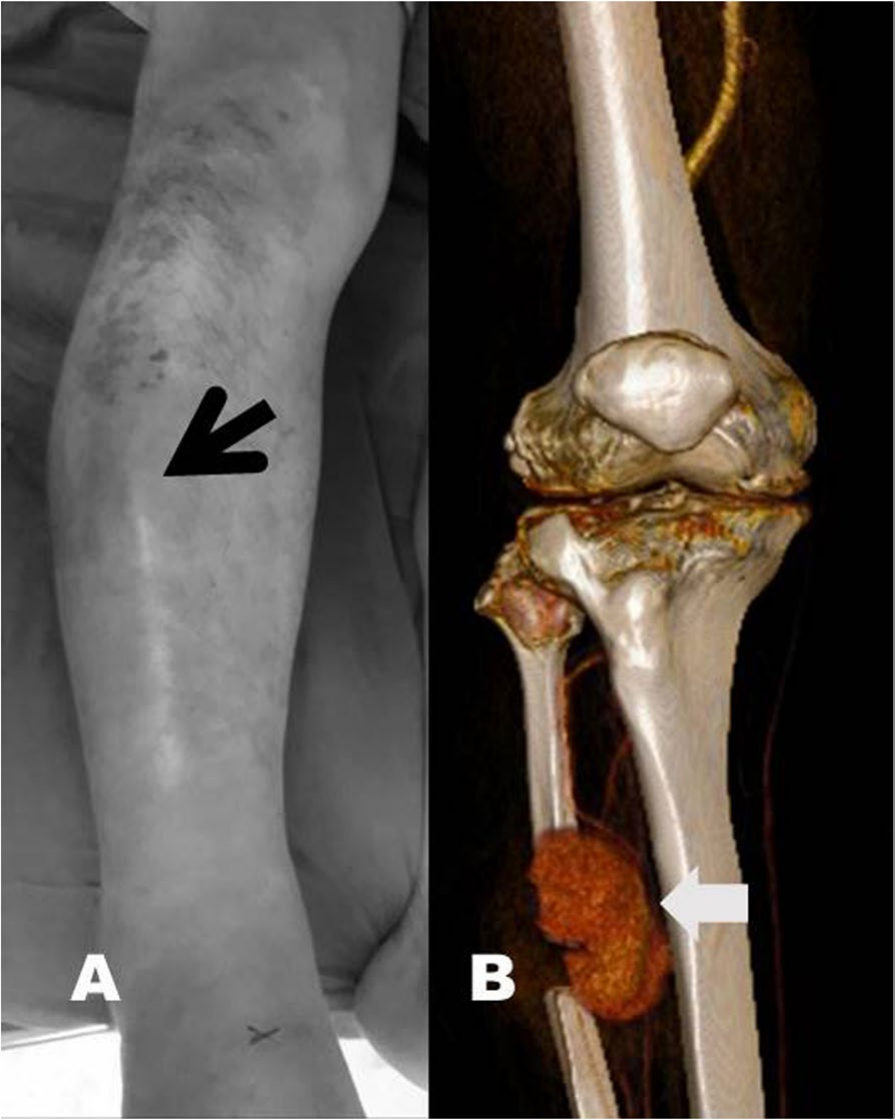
A Clinical photo at emergency room; surrounding ecchymosis and swelling (black arrow). B Reconstructed computed tomography angiography; an out-pouching psuedoaneurysm (white arrow) aries from the anterior tibial artery
